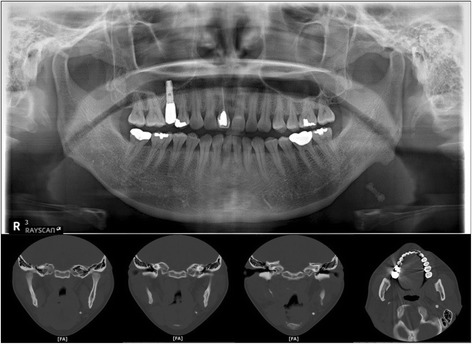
The panoramic radiograph showed an elongated radiopaque structure imposed on the *left* submandibular area (*top*). Computerized tomographic (CT) scan of the mandibular region showed the presence of multiple high attenuated materials and elongated sialolith located within the *left* Wharton’s duct. Very severe atrophic submandibular gland was also found (*bottom*).
